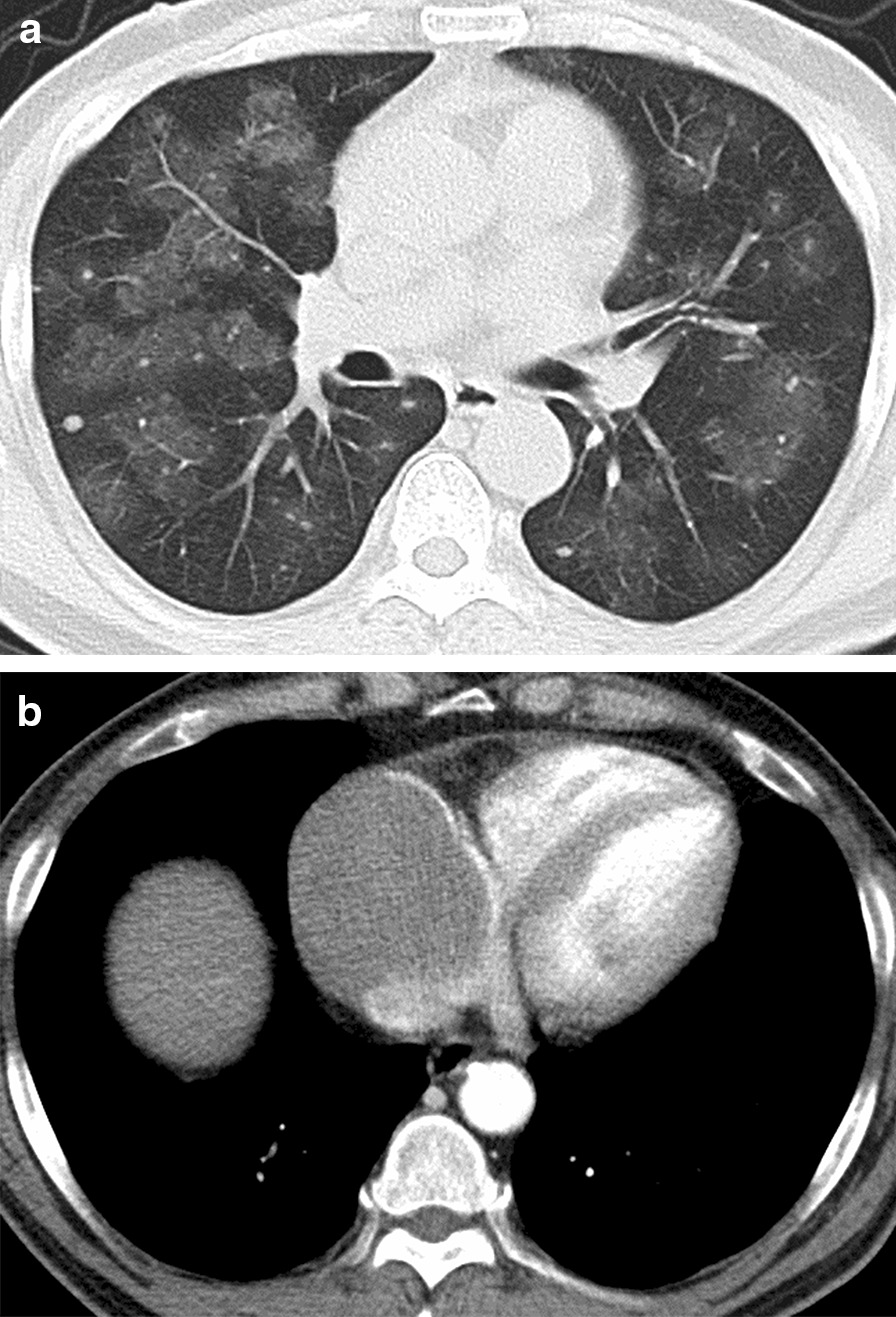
Chest CT. a Multiple parenchymal nodules in lungs surrounded by ground-glass opacity, b a mass (58 mm × 41 mm) located at the right atrium with a clear boundary
